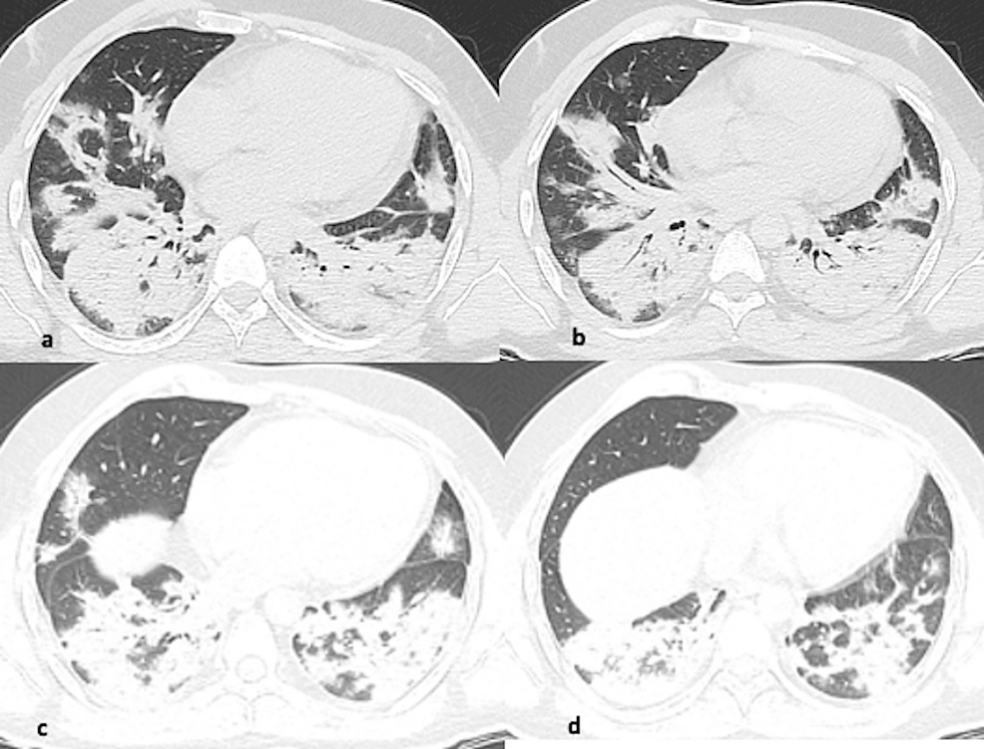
(a-d) Axial CT images from different known COVID-19 patients showing pattern of findings (arrows). Confluent airspace consolidation with air-bronchogram in both the lungs, is more predominant in lower lobes and peribronchial distribution. Areas of reverse halo sign are also present. The appearance resembles diffuse alveolar edema, hemorrhage, or organizing pneumonia.
